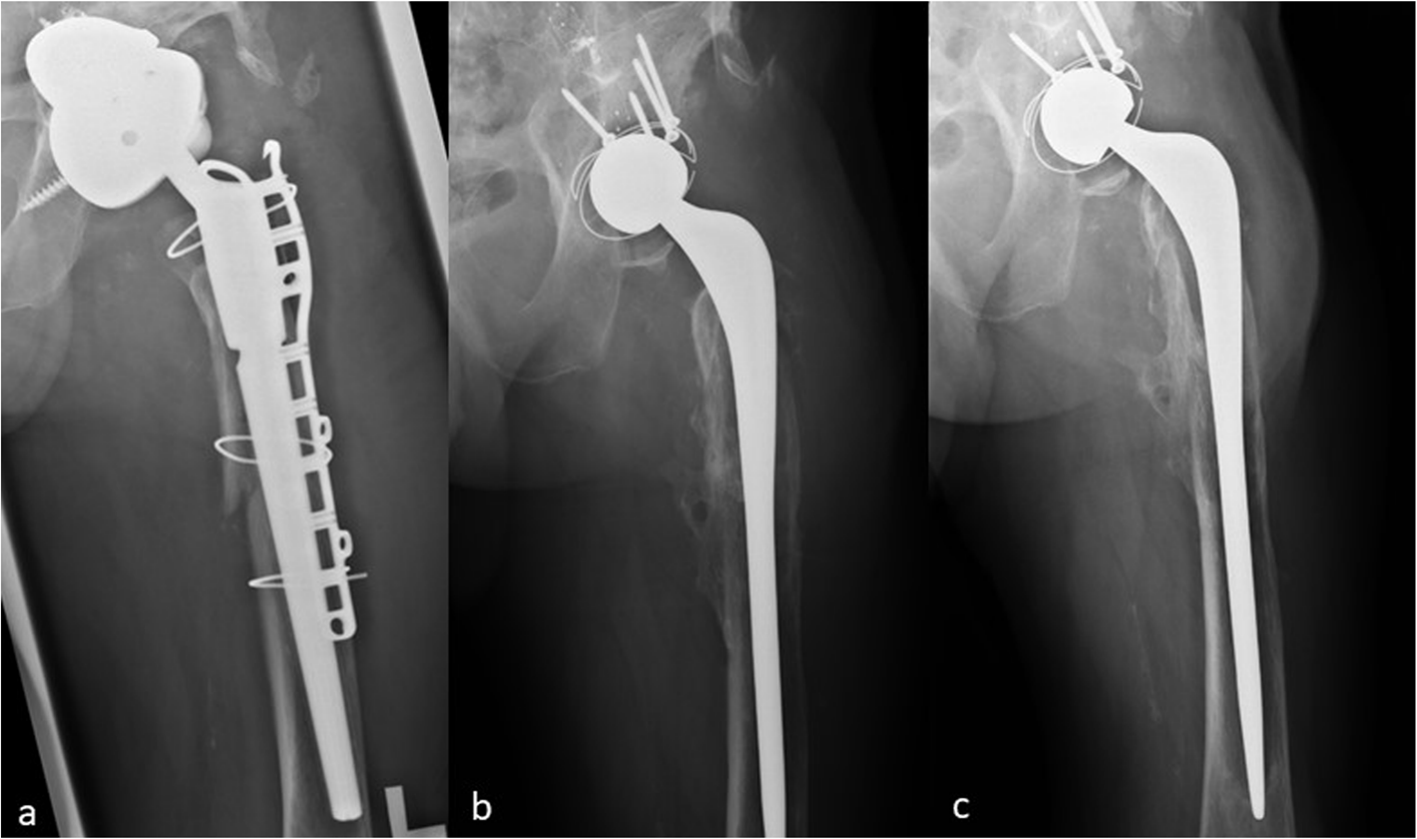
a Pre-operative Anteroposterior (AP) radiograph showing a modular fluted revision femoral stem and cup-cage acetabular construct which were proven to be infected by tissue diagnosis (b) AP radiograph after 1 year of explantation of infected prothesis and treatment with a long CUMAR technique (c) AP radiograph 4.5 years post-operatively demonstrating continued survival of the 1st stage with complete eradication of the infection
